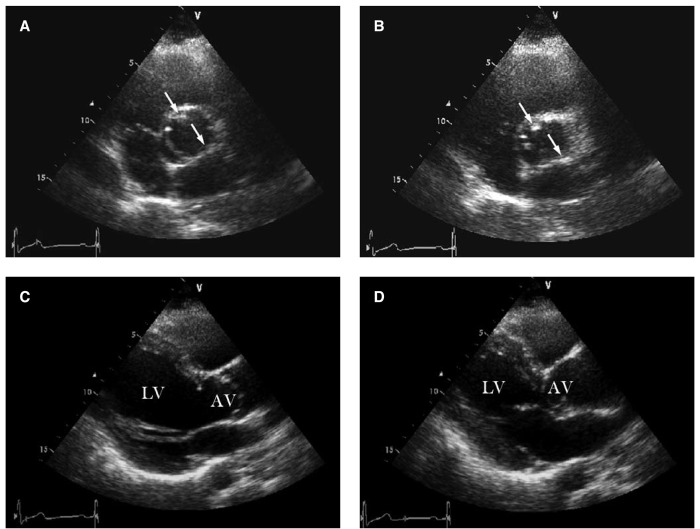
Transthoracic echocardiaography showing a unicuspid aortic valve with a raphe at the 11 o’clock position (upper arrow) and a clear commissure at the 4–5 o’clock position (lower arrow) on a short-axis view during systole (A), and diastole (B). The aortic valve in an integral movement and in a dome-shaped configuration during systole (C) and diastole (D), and left ventricular hypertrophy and dilated aortic root extending 3.8 cm in diameter could be seen from the parasternal long axis view (C, D). AV : aortic valve; LV : left ventricle.
